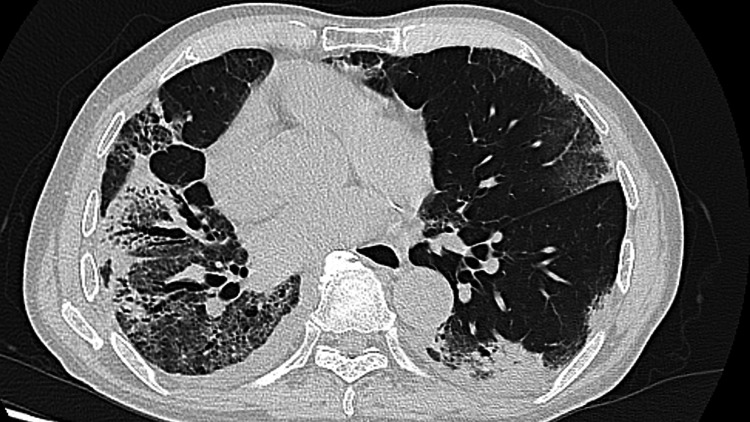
Chest CT on readmission showing diffuse bilateral ground-glass opacities with traction bronchiectasis.
